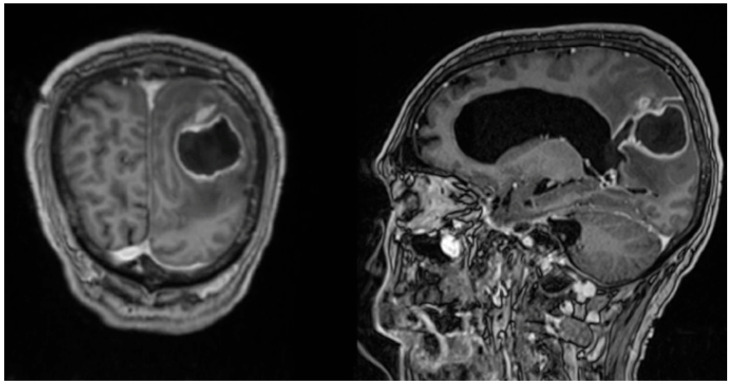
Magnetic Resonance Image of the brain showing left-side parietooccipital abscess in the study patient: horizontal view (left) and lateral view (right).
